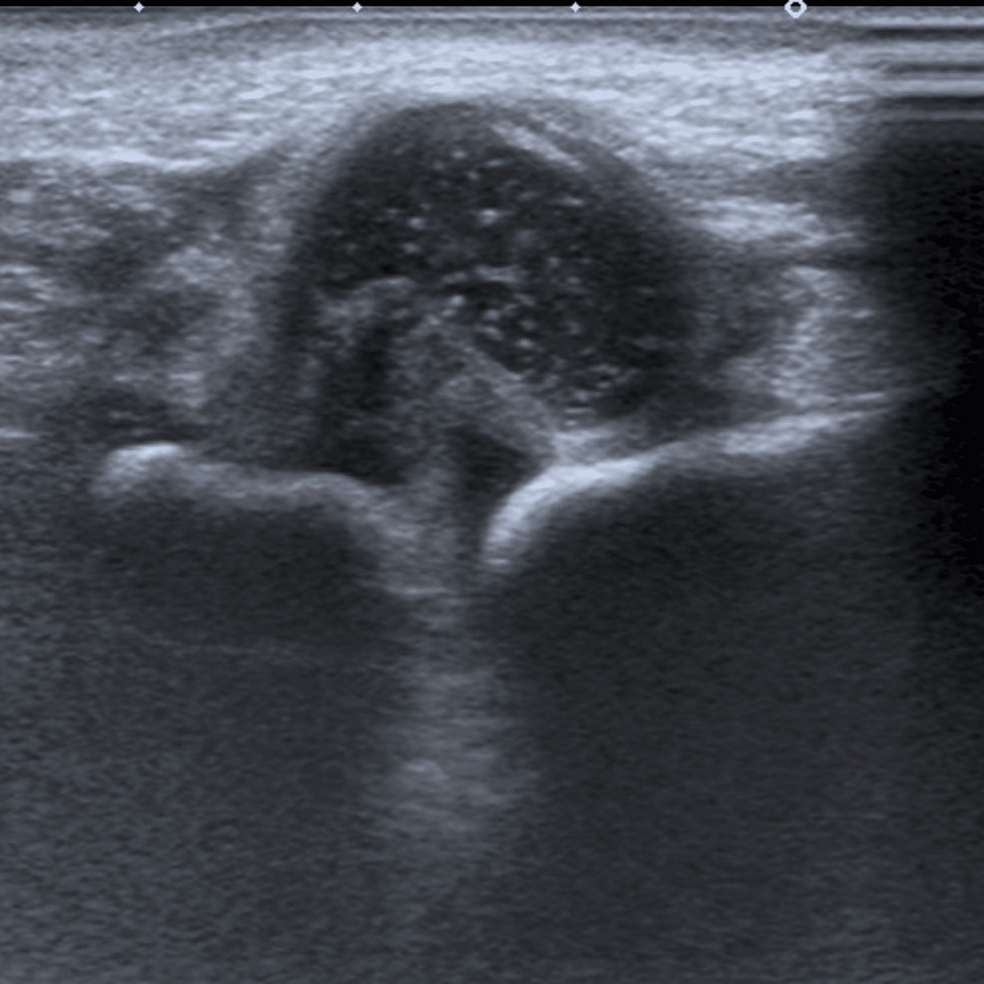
Ultrasound right hip at birth showing right hip dislocation
